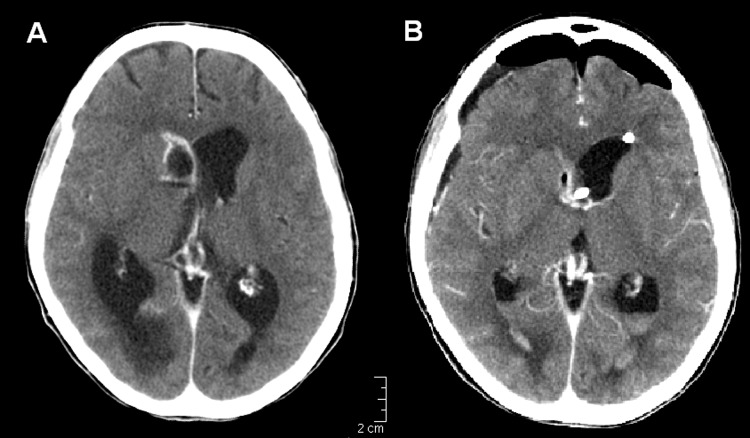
Brain abscess caused by Scedosporium apiospermum (patient 2). A) Images from contrast-enhanced computed tomographic scan show a ring-enhancing lesion in the head of the nucleus caudatus (2 × 1.5 cm) bulging in the right lateral ventricle with concomitant aresorptive hydrocephalus. B) Control computed tomographic scan after surgical drainage and placement of ventricle-peritoneal drainage.
